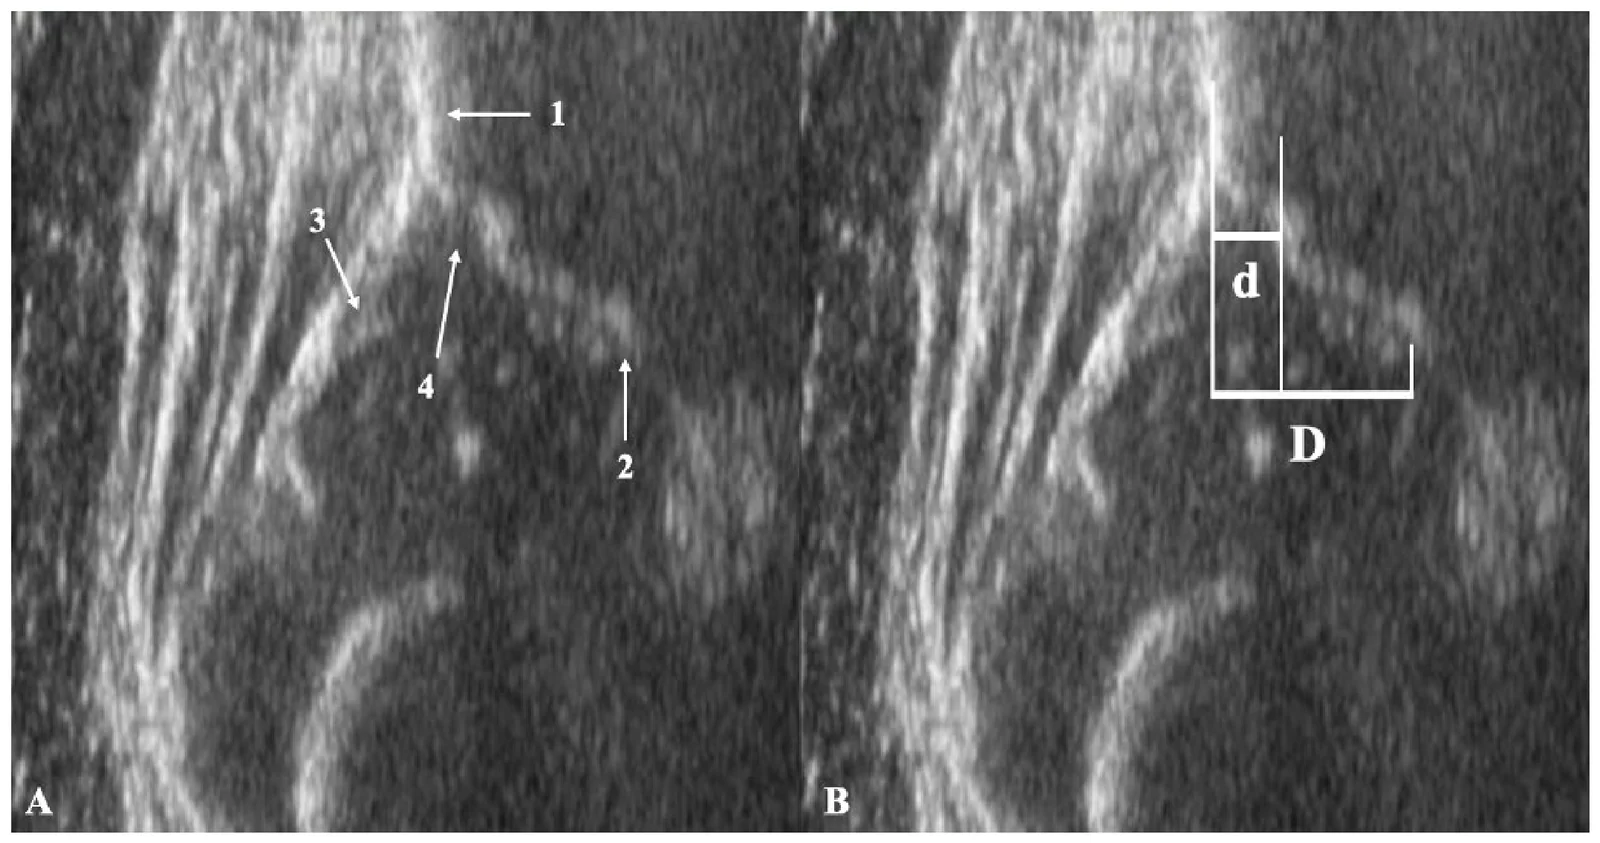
(A,B): Ultrasound image of the right hip in the standard plane, defined by a straight line passing through the iliac crests (1), the inferior border of the ilium (2), and the labrum (3). A SARI is visible (4) (A). To measure the size of the SARI, the width of the SARI (d) is compared with the total width of the bony coverage of the femoral head (D). SARI size = d/D (B).
